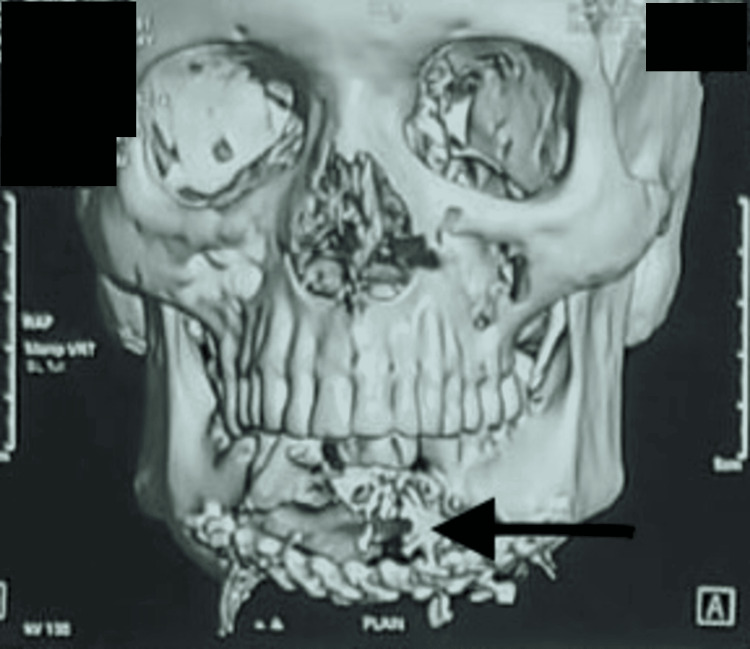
Three-dimensional reconstructed CT scan of the facial skeleton depicting an extensive, expansile, and destructive lesion involving the mandible with cortical perforation and irregular resorption (arrow).
